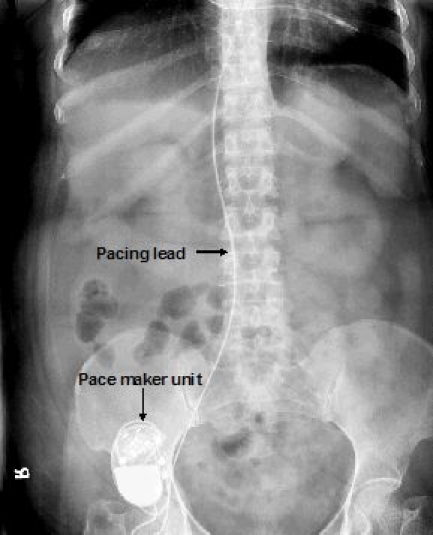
X-ray in anterioposterior view showing pacemaker unit lying under rectus sheath with pacing lead going through external iliac vein to inferior vena cava. Pacemaker unit is looking lateral and down  because of patulous abdomen and X ray has been taken in erect posture.
